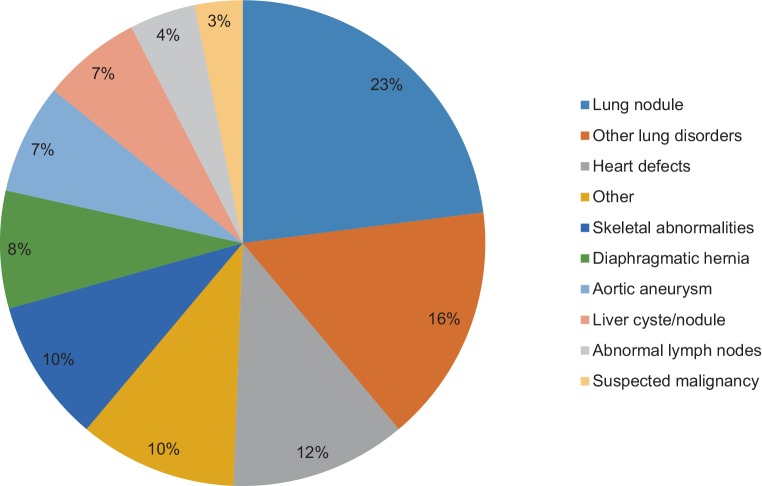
Accidental findings with computed tomography angiography
